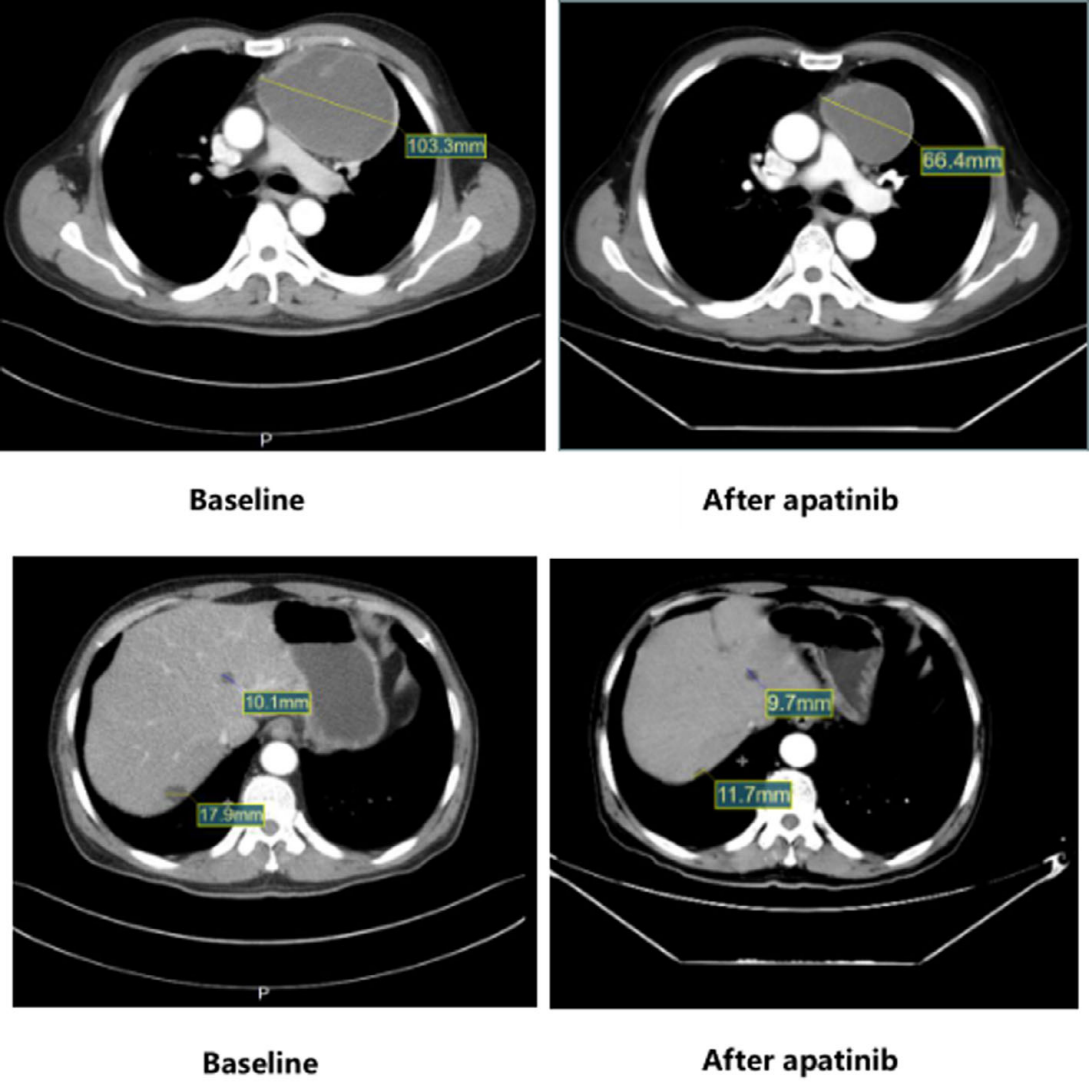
Representative pretreatment enhanced computed tomography images (left row) and post‐treatment images (right row) are shown for a 63‐year‐old man diagnosed with stage IVB thymic carcinoma with liver metastases. After 19 months of second‐line apatinib single‐agent treatment, the size of the primary lesion was significantly reduced, while the liver metastases remained stable.
